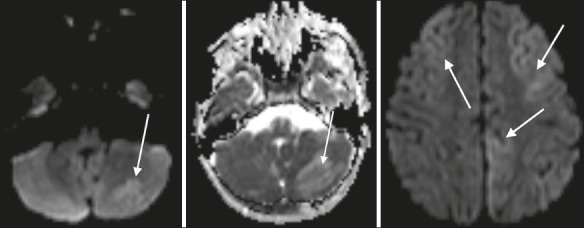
Brain MRI. Axial DWI with apparent diffusion coefficient mapping showing lesions
